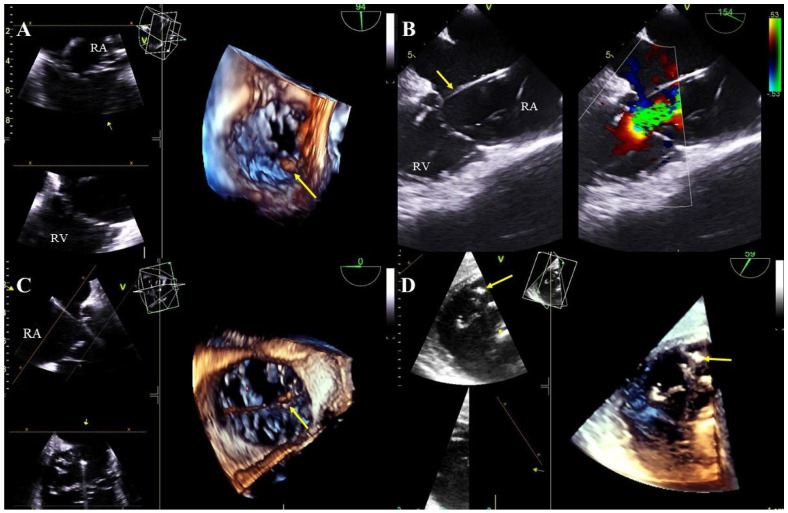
Tricuspid valve dysfunction caused by the presence of the electrode (TEE 2D, 3D). The lead (yellow arrow) in the tricuspid valve supports the septal leaflet and hinders proper coaptation of the leaflets (TEE 3D) (A,C). Severe tricuspid valve regurgitation resulting from the septal leaflet being pathologically supported by the lead (yellow arrow), (2D, color Doppler image from panel A) (B). The posterior leaflet of the tricuspid valve is perforated by the lead (yellow arrow) (D).
